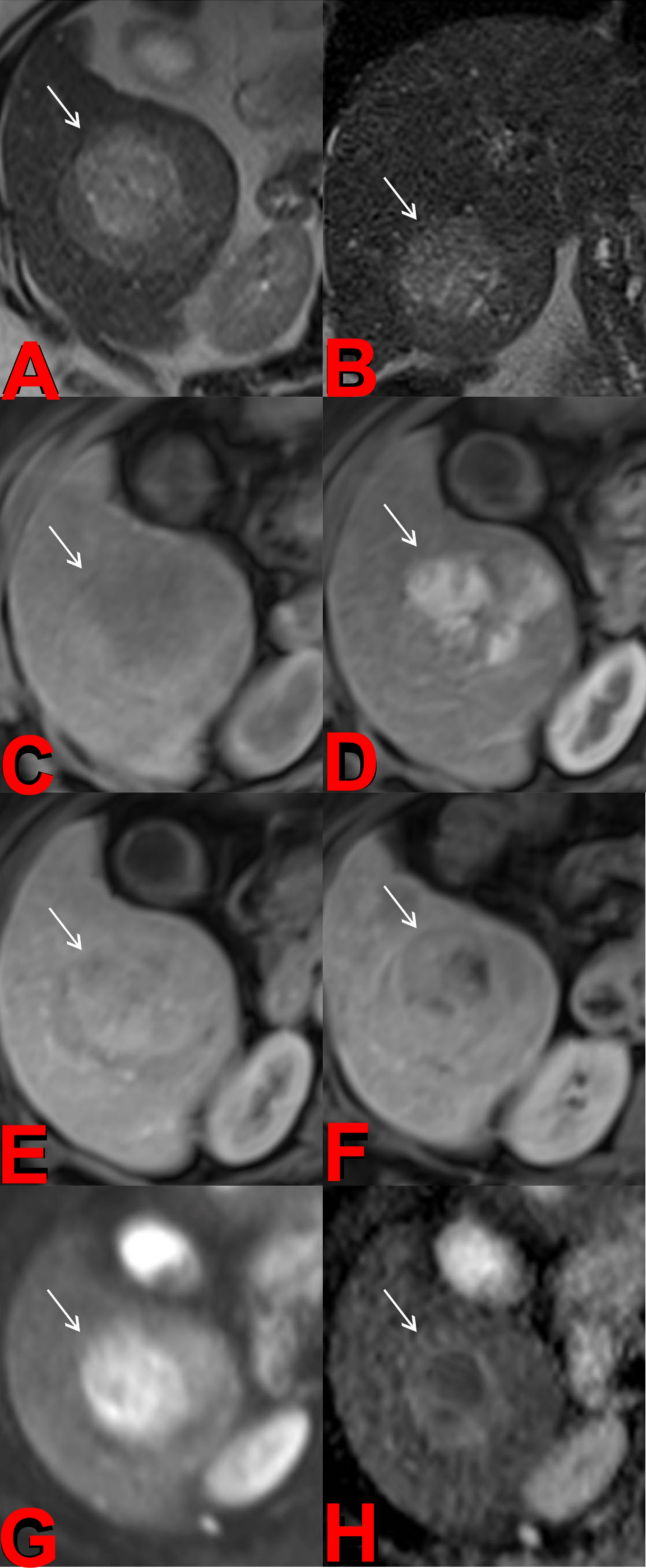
Pretreatment Magnetic Resonance Imaging (MRI) Axial (a) and coronal (b) T2-weighted images of the liver demonstrate a mildly hyperintense mass in the right hepatic lobe. Precontrast (c), arterial (d), portalvenous (e), and delayed (f) postcontrast T1-weighted images demonstrate heterogeneous early arterial hyperenhancement and subsequent portalvenous / delayed washout of the mass compatible with hepatocellular carcinoma. The diffusion weighted imaging (DWI) (g) and its corresponding apparent diffusion coefficient (ADC) map (h) demonstrate foci of restricted diffusion within the tumor.
